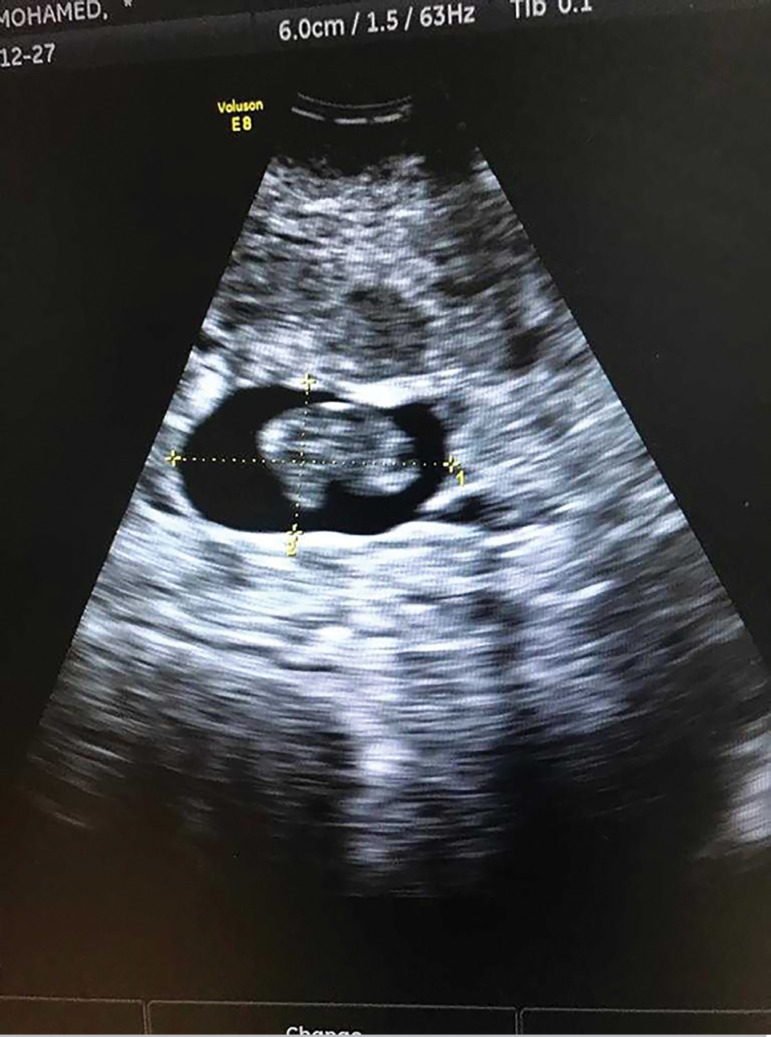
Ultrasound picture showing pregnancy sac with fetal pole
